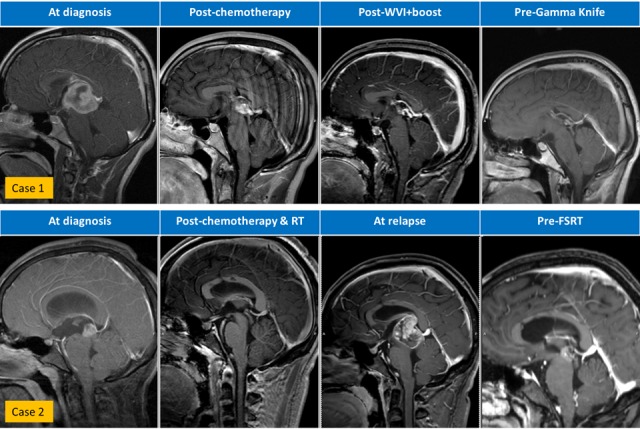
Serial sagittal T1-weighted MRI brain scans with gadolinium WVI = whole ventricular irradiation; CSI = craniospinal irradiation; FSRT = fractionated stereotactic radiotherapy; RT = radiotherapy
